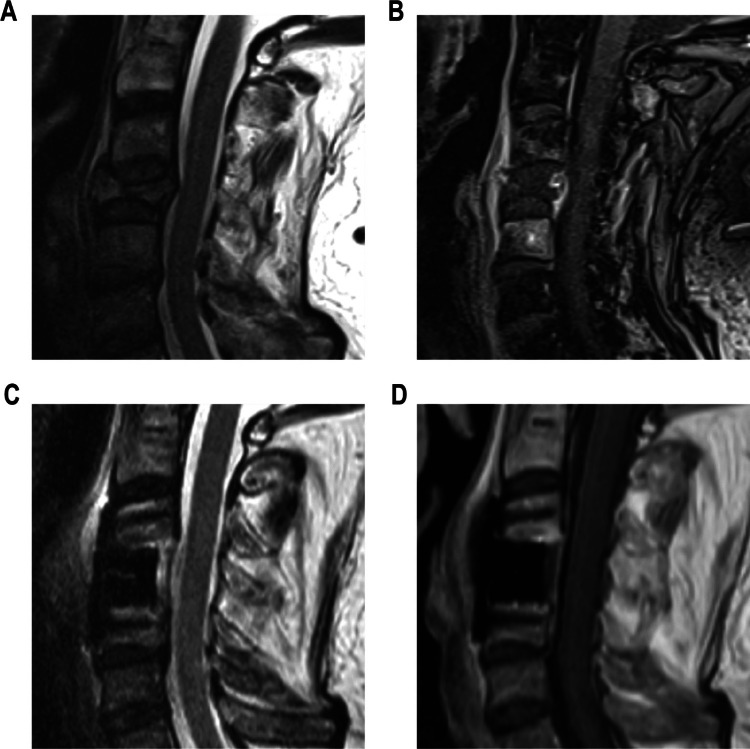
Case 2: Pre- (A, B) and postoperative (C, D) sagittal MRI T2 (A, C) and contrast enhanced T1 weighted imagesi (B, D)
